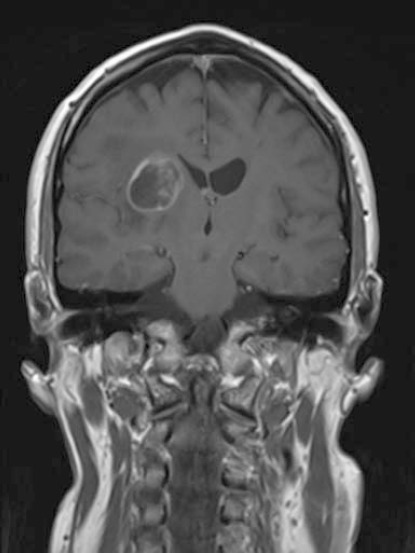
MRI (T1-weighted with gadoliunium) at presentation in April 2008.
